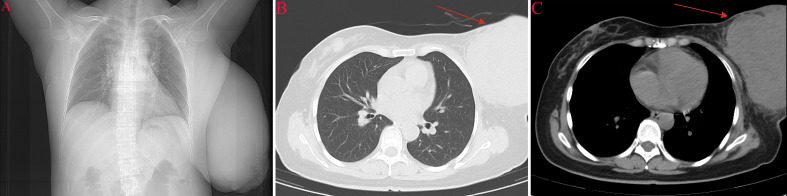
Preoperative chest imaging (A–C). (A) Chest radiograph shows no obvious pulmonary metastasis or pleural effusion. (B) Axial CT (lung window) demonstrates a large left breast/anterior chest wall mass (arrow) without intrathoracic metastatic lesions. (C) Axial CT (mediastinal window) shows the mass with adjacent soft-tissue involvement (arrow).
